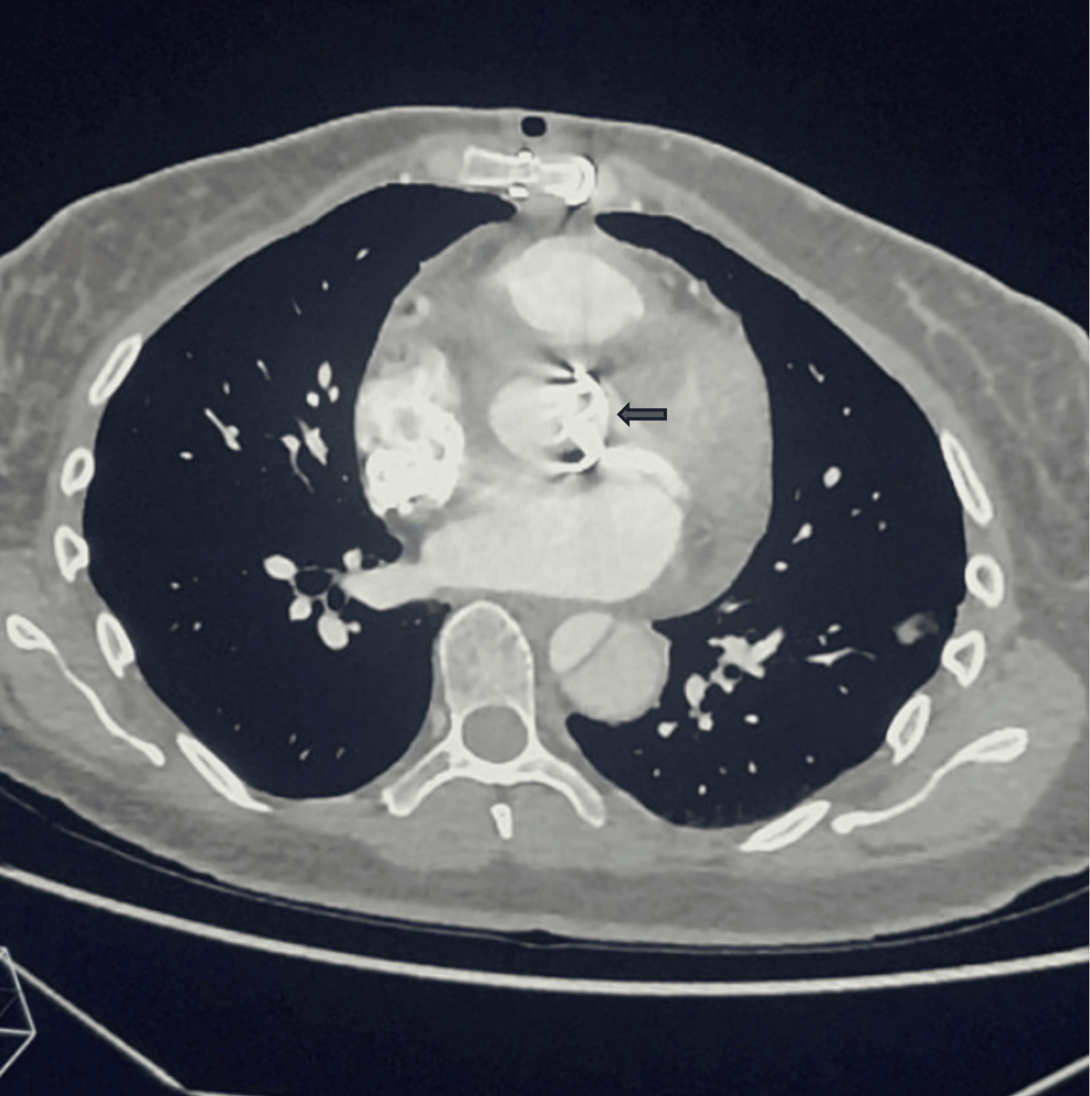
Follow-up CT scan. A properly positioned mechanical aortic prosthesis is observed (arrow).
